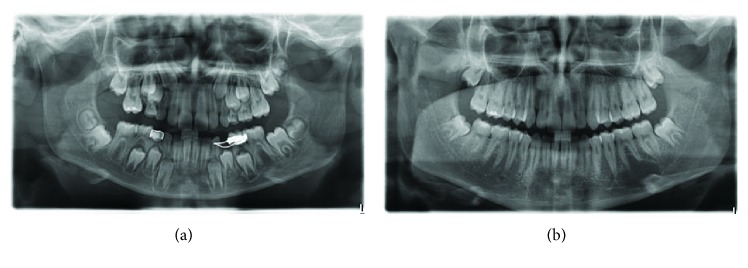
(a) Orthopantomography in the phase of mixed dentition. A normal rate of tooth eruption, without any radiolucent lesion, is seen in the area of the third quadrant. (b) Follow-up orthopantomography of the third molars after orthodontic treatment. The radiolucent lesion affecting the areas of 35, 36, and 37 is diagnosed.
